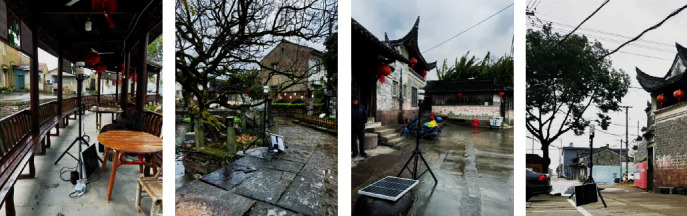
Location of ultrasonic integrated weather stations. (a) Measuring point 1. (b) Measuring point 2. (c) Measuring point 3. (d) Measuring point 4.
